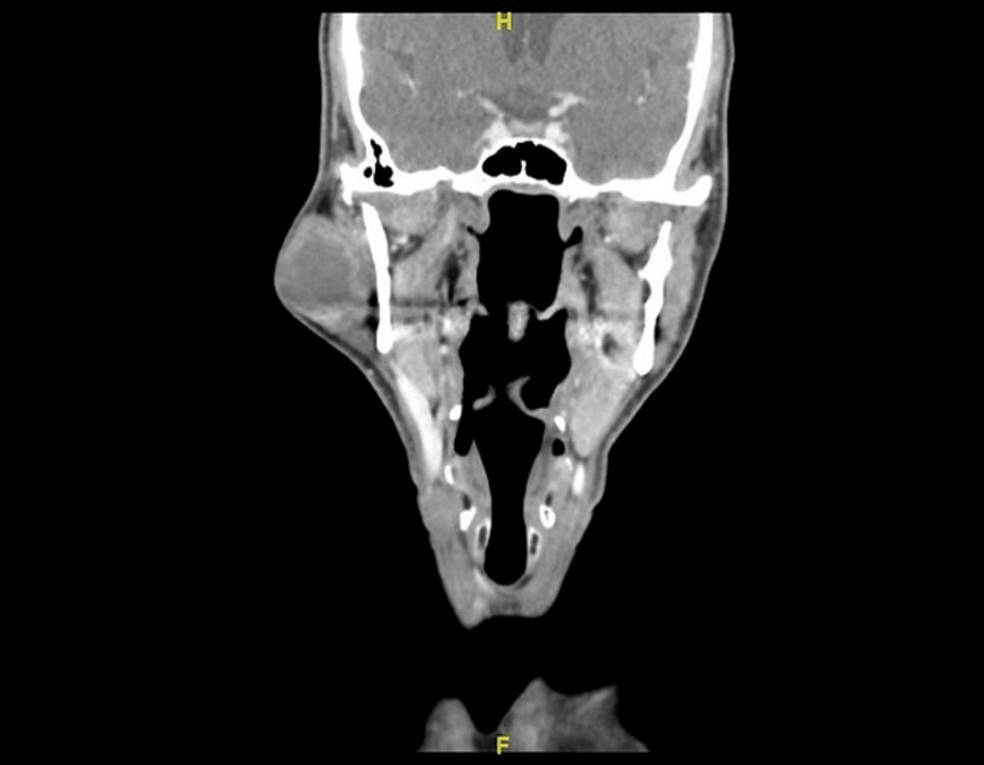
Coronal contrast-enhanced CT soft tissue window of the head showing a mass of soft tissue density in the superficial lobe of the right parotid gland.
